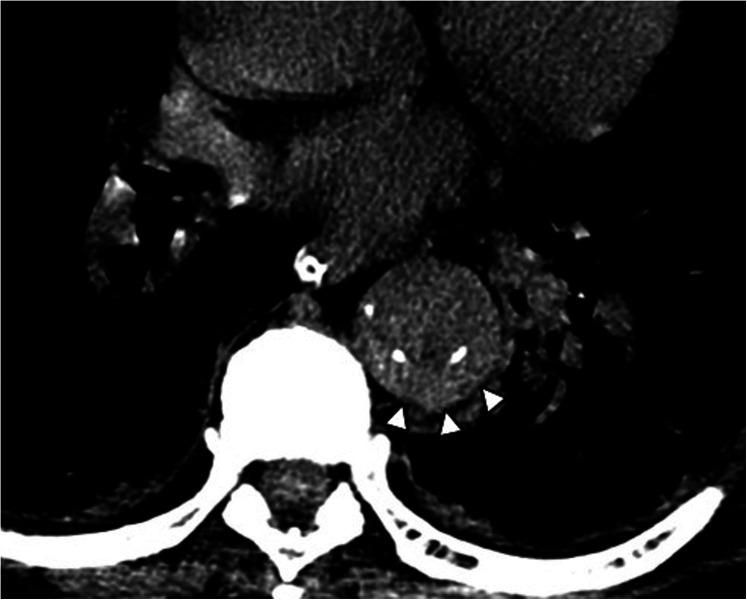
Non-contrast CT axial image in a 73-year-old female patient shows the diagnostic hallmark of IMH: displacement of intimal calcifications and hyperattenuating crescentic thickening of the aortic wall (arrowheads).
